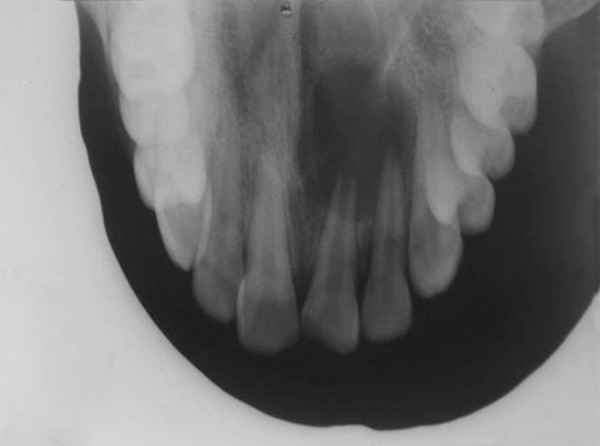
Preoperative diagnostic X-ray
